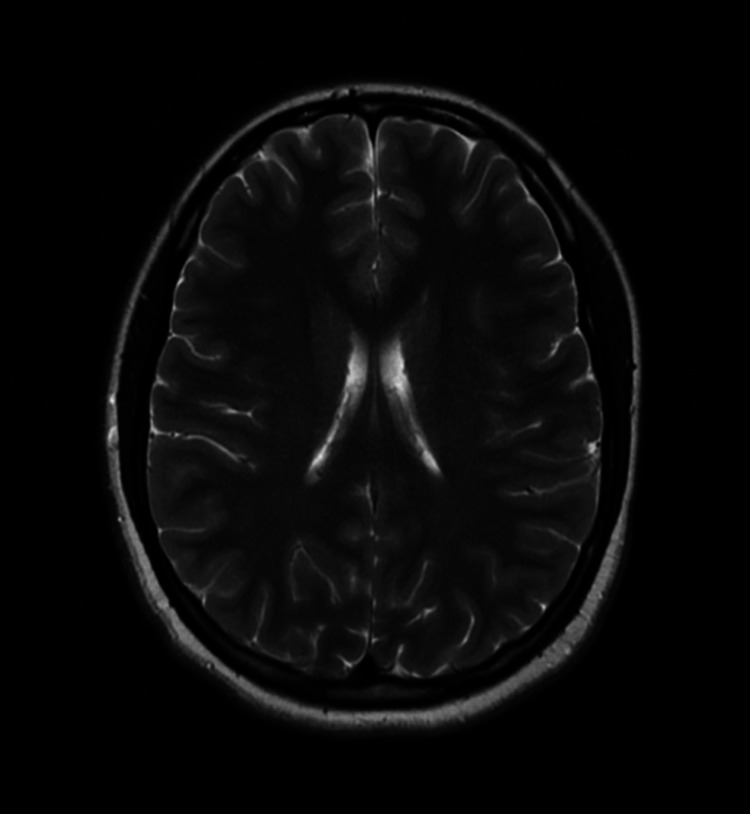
Normal MRI (axial T2) Case courtesy of Associate Professor Frank Gaillard, Radiopaedia.org, rID: 37605
